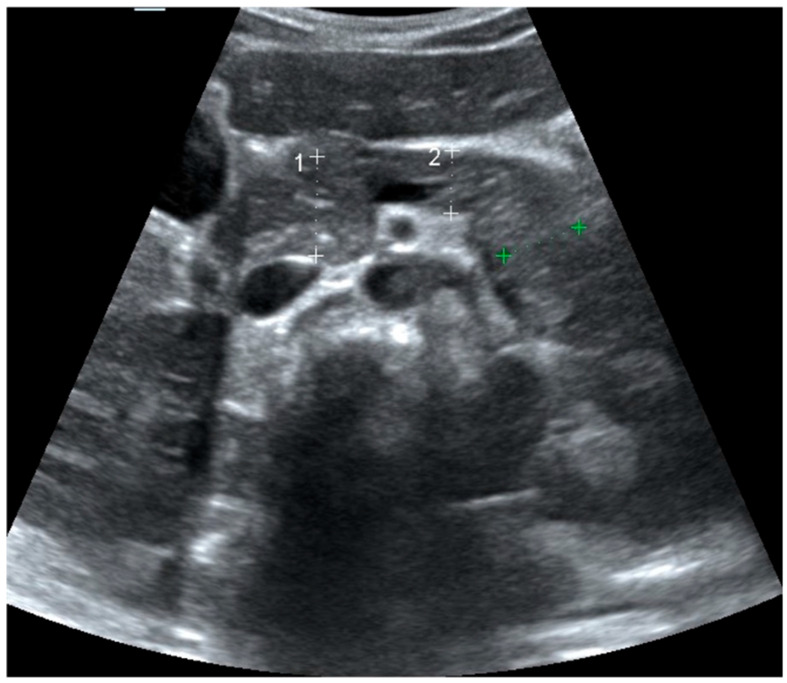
Upper abdominal ultrasound. Pancreatic size measurements: 1 = head, 2 = body; tail shown in green. For each region, the measurement corresponds to the dotted line between the two “+” caliper marks—white “+” for head (1) and body (2), green “+” for tail.
